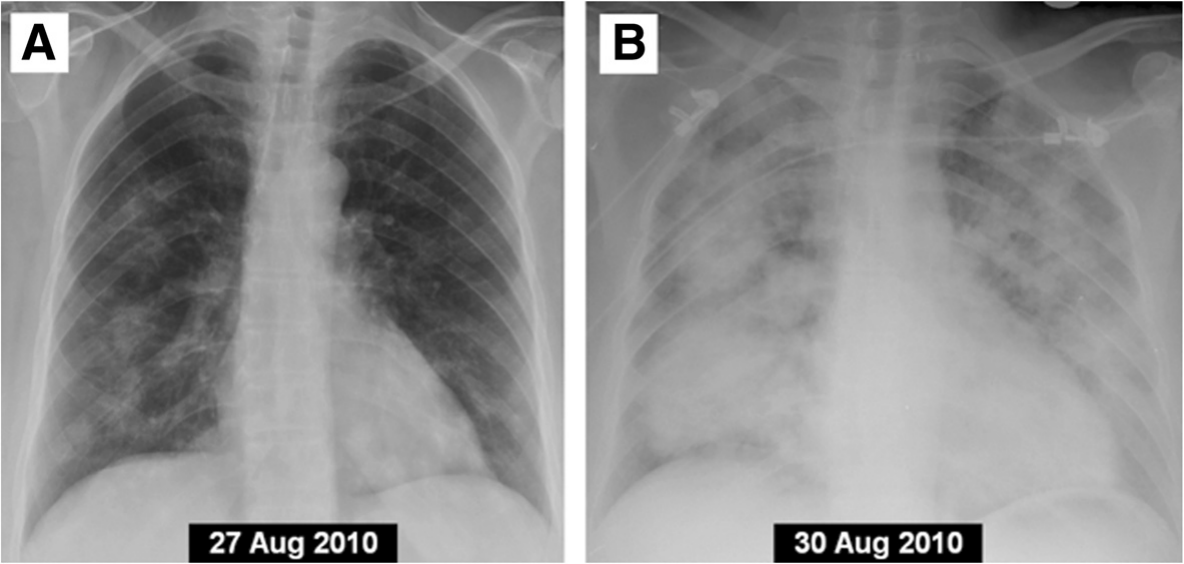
Posterior-anterior chest X-ray 4 days prior to admission. (A) Showing bilateral infiltrates. (B) Repeat chest X-ray on admission showing significant interval change consistent with diffuse alveolar hemorrhage.
